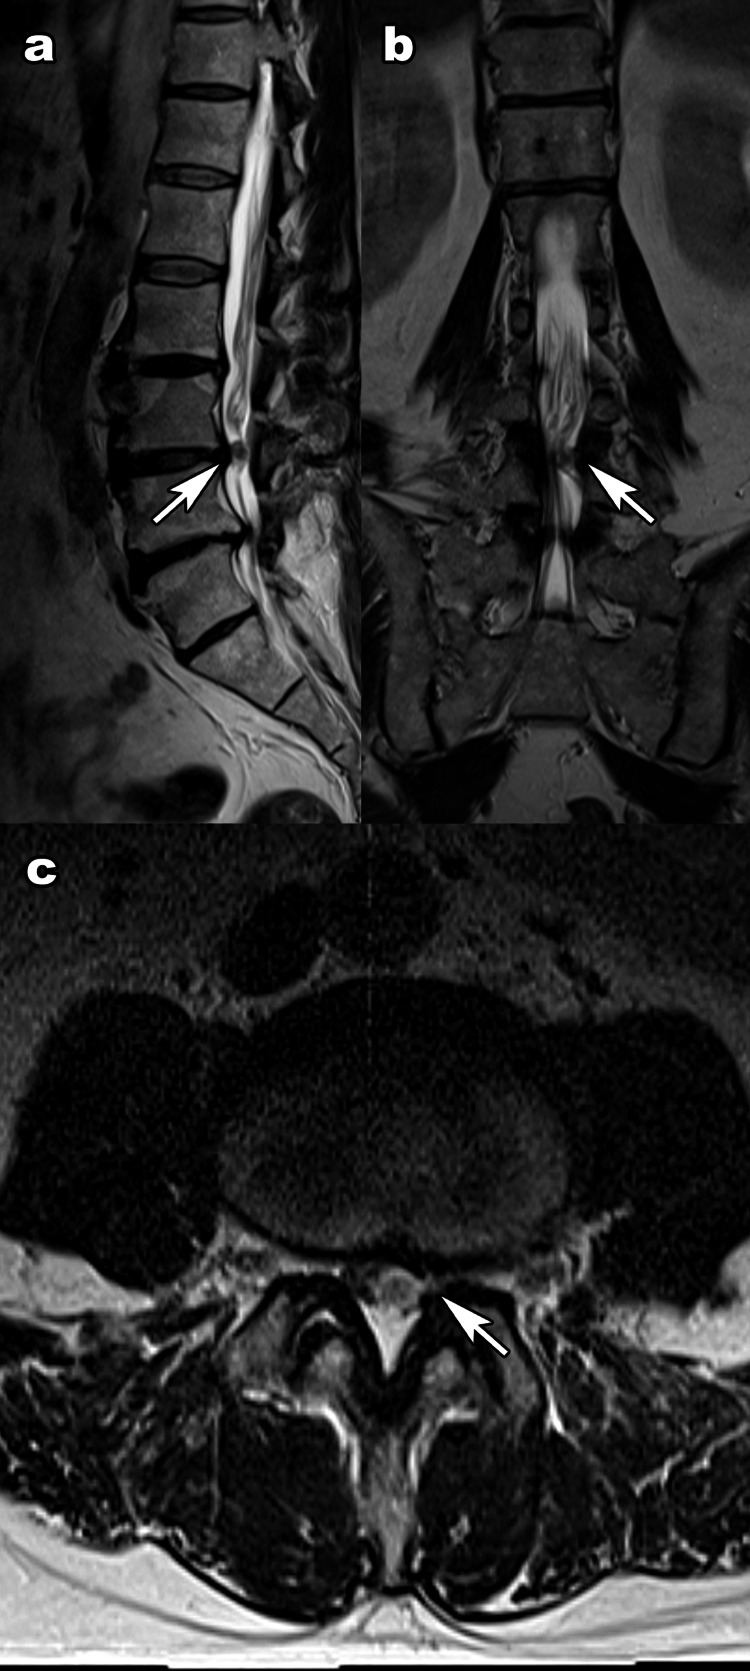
Initial magnetic resonance imaging. T2-weighted images suggested an intradural lesion at the L3-L4 level. (a) Sagittal images showed no obvious discontinuity of the posterior longitudinal ligament (arrow). (b) Coronal images showed an unclear relationship between the lesion and the dura (arrow). (c) In retrospect, this transverse image suggested lateral dural discontinuity (arrow).
